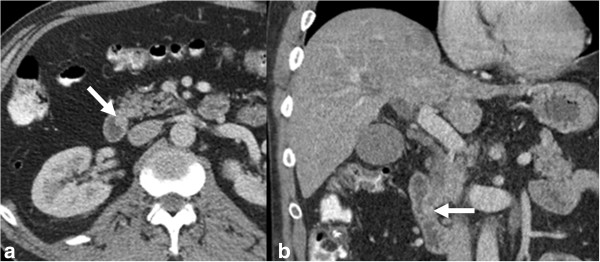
Ampullary carcinoma. Contrast enhanced axial CT (a) and coronal reformat (b) showing a small polypoid mass (arrow) representing carcinoma in the ampulla of the bile duct.
